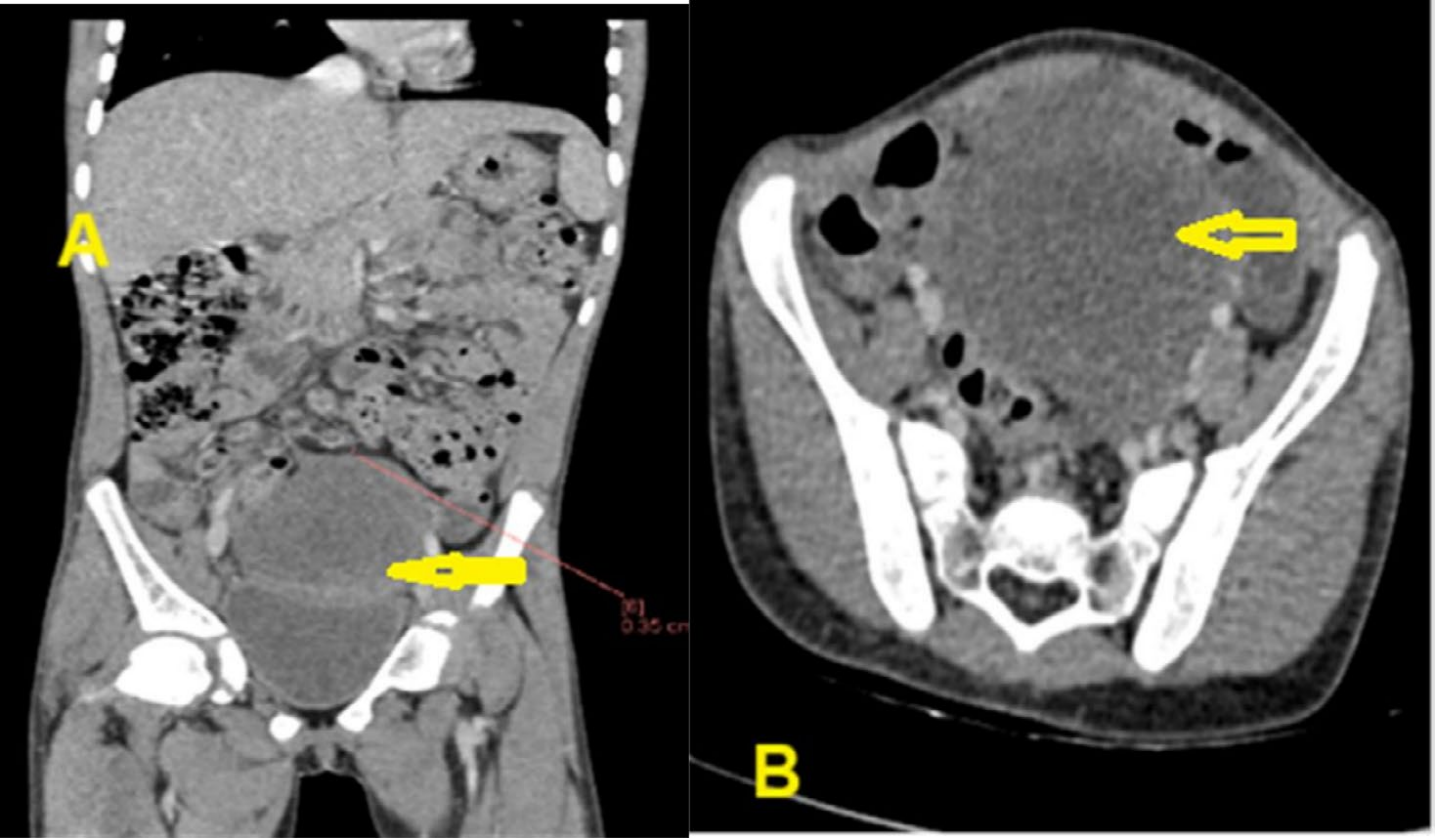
Abdominal CT scan with contrast (A) coronal and (B) axial view revealed significantly reduced in size to 5 × 4 × 5 cm after 5 cycles of chemotherapy, and there was no suspicious nodule for pulmonary metastases.
